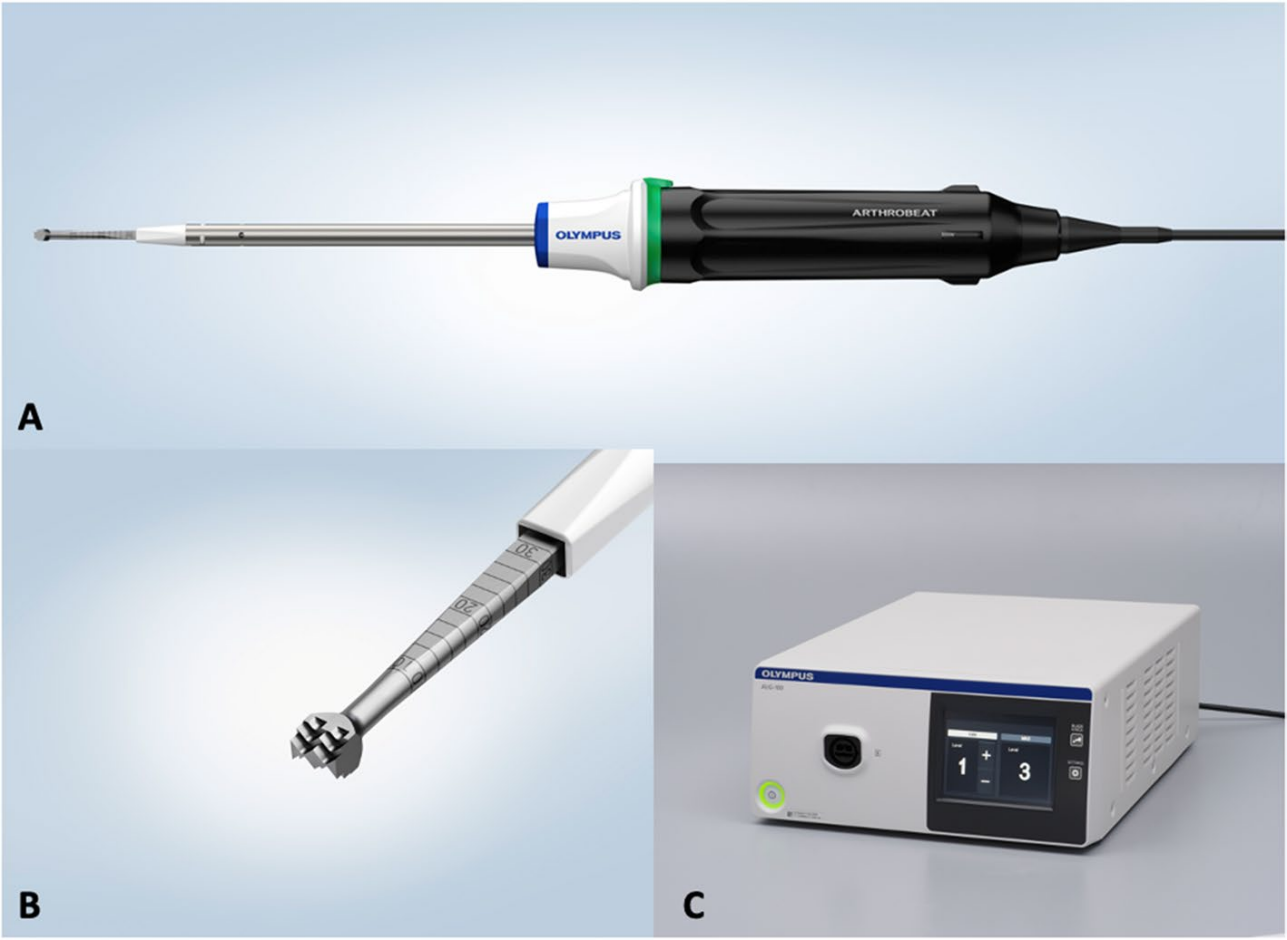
Arthrobeat ultrasound device. A: Arthrobeat Transducer; B: Arthrobeat 4 × 5 mm rectangle blade; C: Arthroscopic ultrasonic generator (AUG-100)
